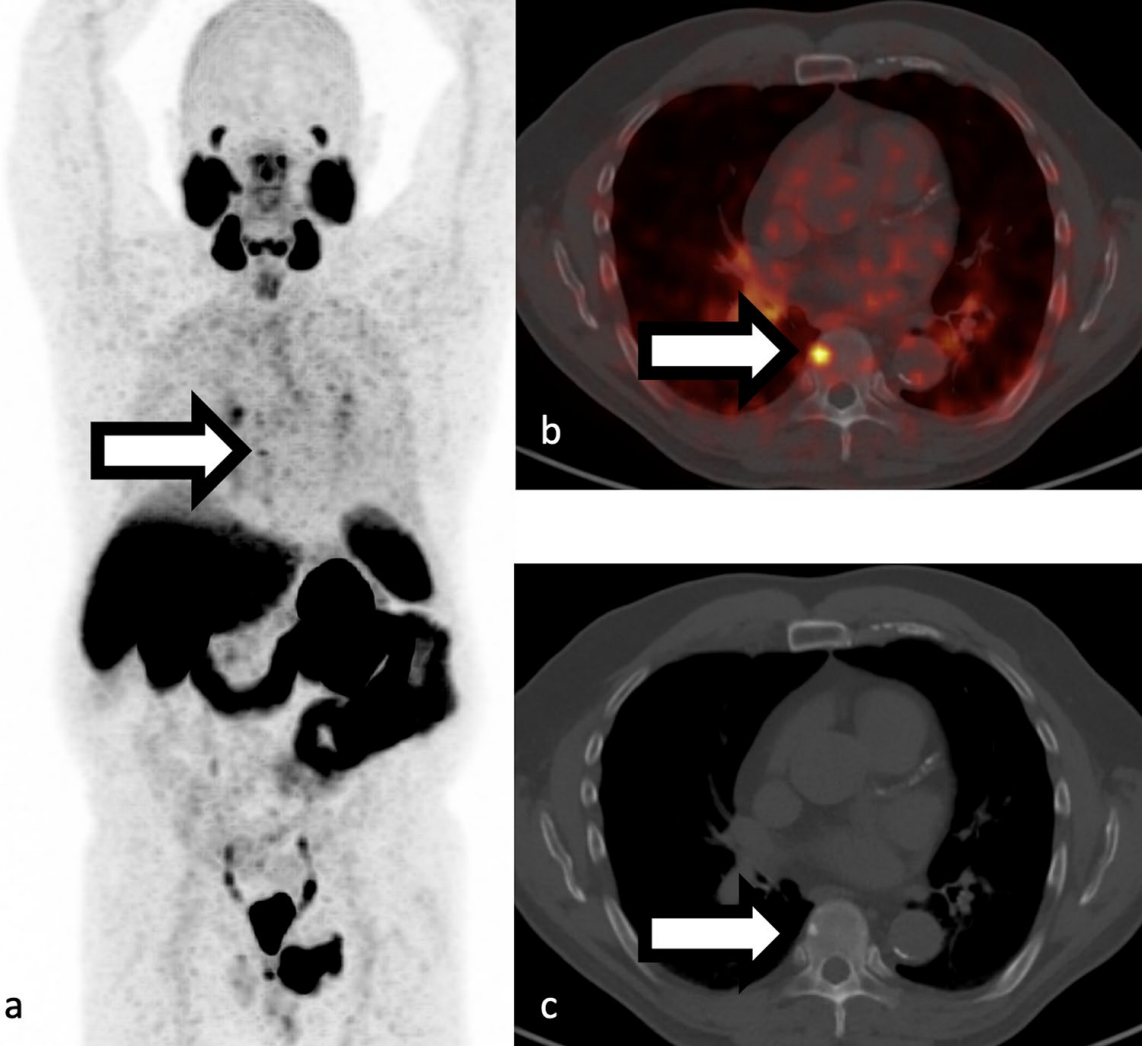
a–c Example of oligometastatic osseous disease in a 68-year-old man with history prostatectomy now presenting with biochemical recurrence and PSA of 1.3 ng/mL. 68 Ga-PSMA-11 PET/CT showed a single focus of PSMA uptake (white arrow in panels a and b, SUVmax 4) corresponding to a small sclerotic focus in the T8 vertebral body (white arrow in panel c). Activity below bladder corresponds to urinary contamination. Maximum intensity projection a SUV scaled at 0–5
